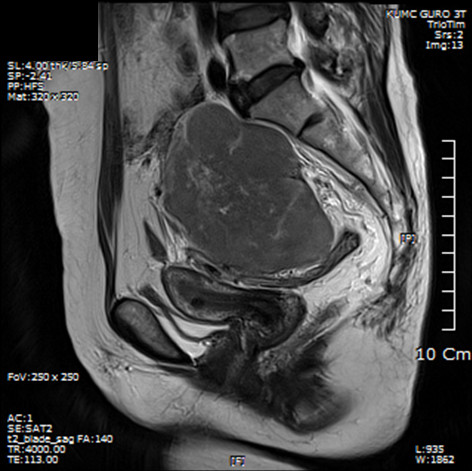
Pelvic magnetic resonance imaging scan of T2-weighted image. The mass shows iso- to slightly low signal intensity with heterogeneous enhancement. The uterus and sigmoid colon were displaced anteroinferiorly.
